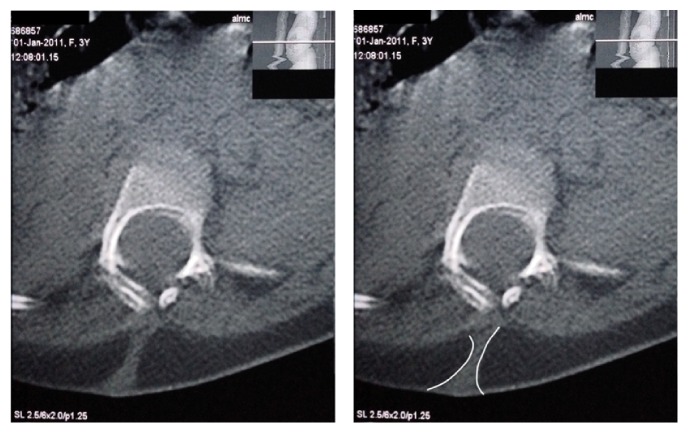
A transverse CT section demonstrating a dorsal dermal sinus connecting the epidermis to the epidural space.
